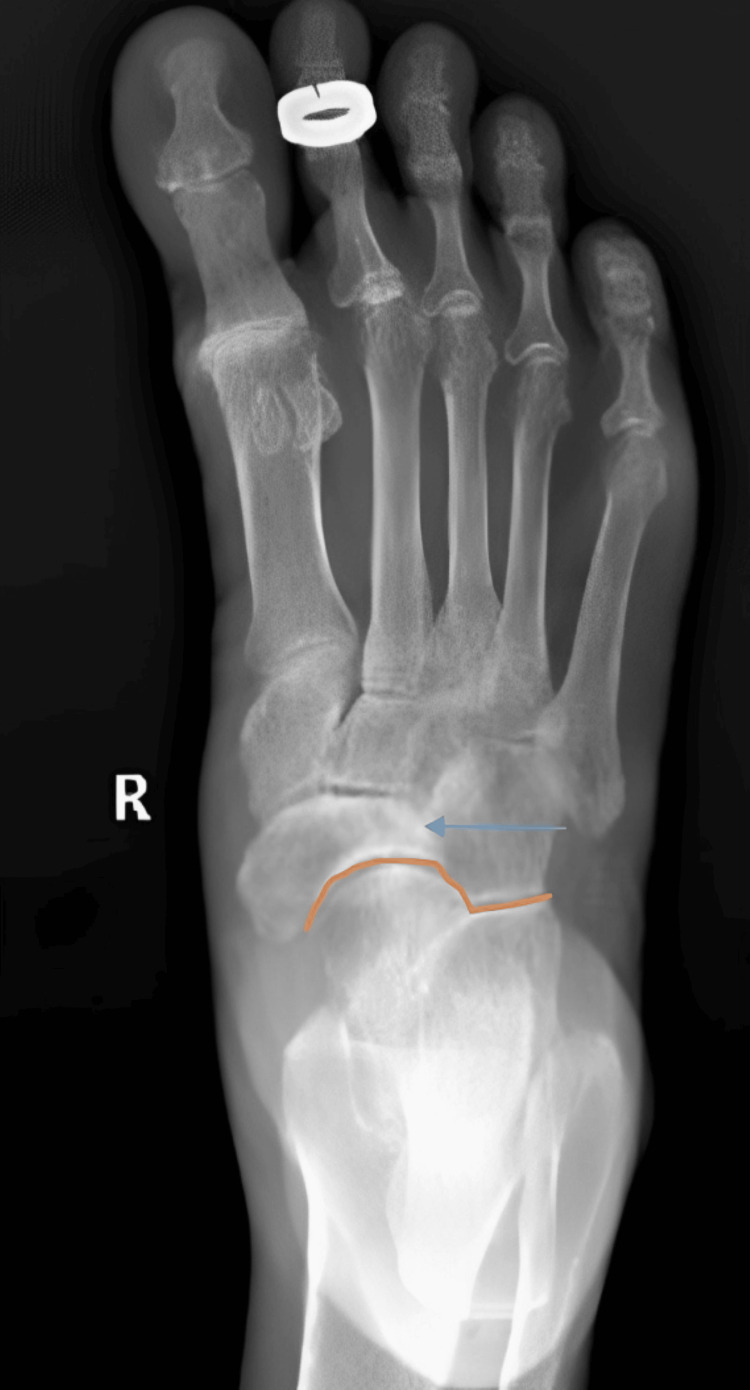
AP radiograph of the right foot showing a partially collapsed and sclerotic lateral half of the navicular bone (blue arrow) and mild lateral subluxation of the talar head with disruption of the cyma line (orange arrow), consistent with Müller-Weiss syndrome
